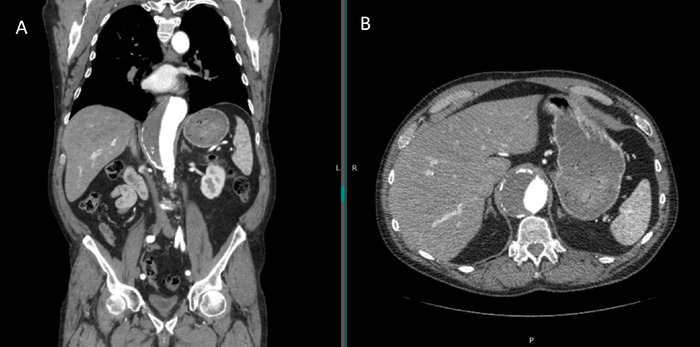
(A) Sagittal and (B) axial computed tomography images show aneurysmal degeneration of the proximal supraceliac and paravisceral abdominal aorta, with a maximum diameter of 5.7 cm at the level of the aortic hiatus (T12 vertebra).
